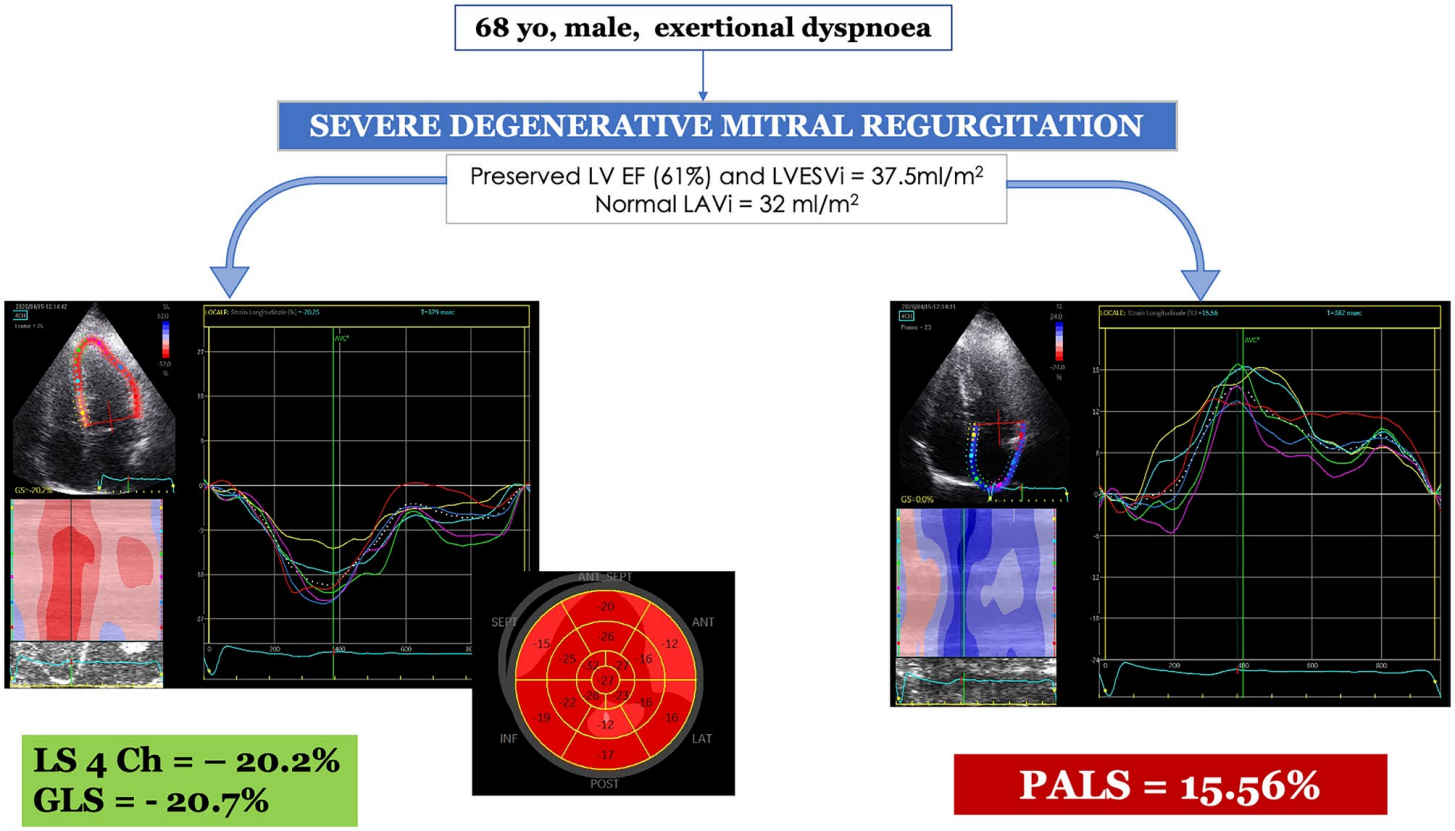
Representative case of a patient with severe mitral regurgitation symptomatic for exertional dyspnea, referred for cardiac surgery. Basic echocardiography showed preserved left ventricular (LV) dimensions and function (LV ejection fraction = 61%, LV end-systolic volume index 35 ml/m^2^) and normal left atrial (LA) volume (LA volume index = 32 ml/m^2^). Interestingly, speckle tracking echocardiography detected left atrial dysfunction (considerably reduced peak atrial longitudinal strain = 15%, *right*) with preserved LV strain (4-chamber longitudinal strain = −20.2%, global longitudinal strain = −20.7%, *left*). GLS global longitudinal strain, LS longitudinal strain, LVEF left ventricular ejection fraction, LVESVi left ventricular end-systolic volume index, PALS peak atrial longitudinal strain
